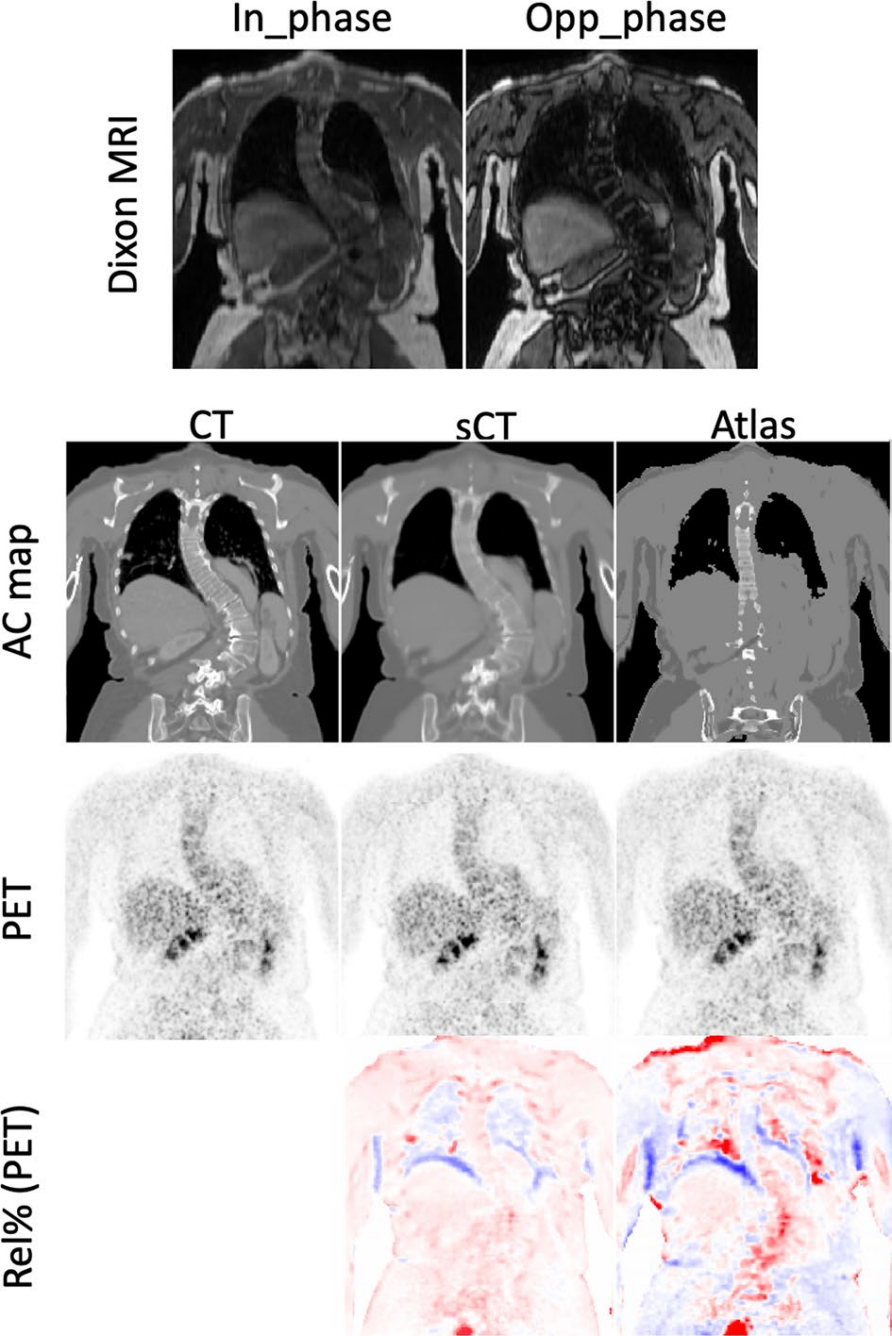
A clinical case with severe scoliosis. Dixon MRI images (first row); Reference CT, sCT, and atlas-based AC maps (second row); corresponding PET image (third row); percentage difference map (last row)
